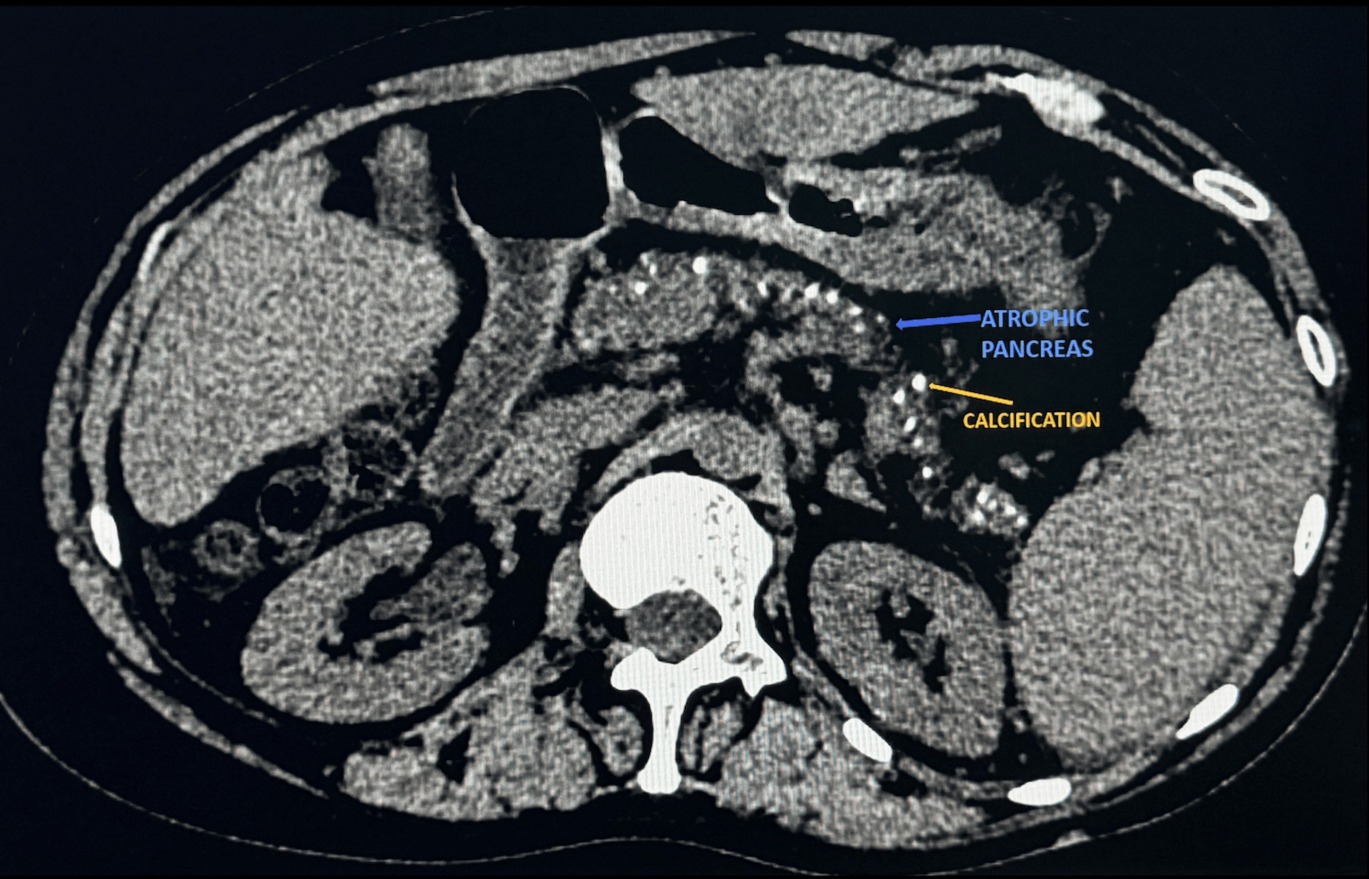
Axial non-contrast computed tomography (NCCT) revealed atrophic pancreas (blue arrow) with specks of parenchymal calcification (yellow arrow) suggestive of chronic calcific pancreatitis Pancreatic atrophy reflects long-standing parenchymal destruction and fibrosis, while coarse parenchymal calcifications (hyper intensities marked by yellow arrow) are characteristic of chronic calcific pancreatitis, resulting from repeated inflammation and ductal obstruction leading to protein plug formation and calcification. These imaging findings corroborate the diagnosis of advanced chronic pancreatitis with exocrine insufficiency.
